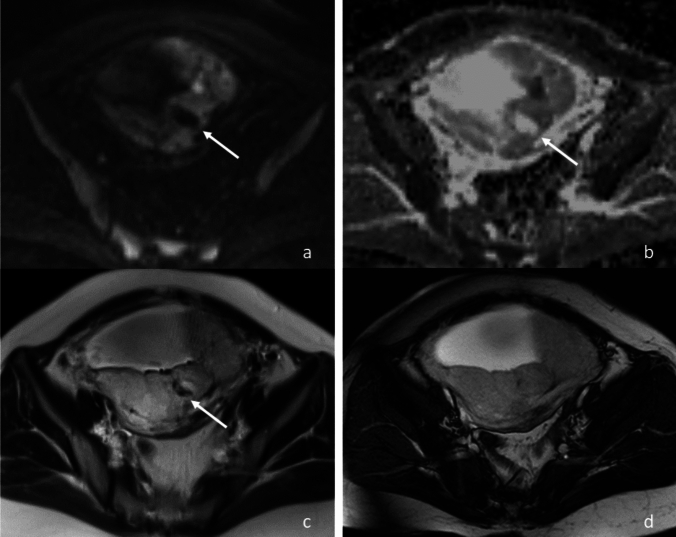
A 30-year-old woman with placenta previa and placenta percreta. a Axial diffusion-weighted image shows a hypointense ovoid space (arrow) in the placenta. b The apparent diffusion coefficient map shows hyperintense space (arrow) corresponding to the hypointense diffusion area in (a), indicating diffusion lacunae. c Axial T2-weighted half-Fourier acquisition single-shot turbo spin echo shows flow void in the diffusion lacuna (arrow). d True fast imaging in steady-state precession shows high signal intensity throughout the placenta, including the diffusion lacuna
